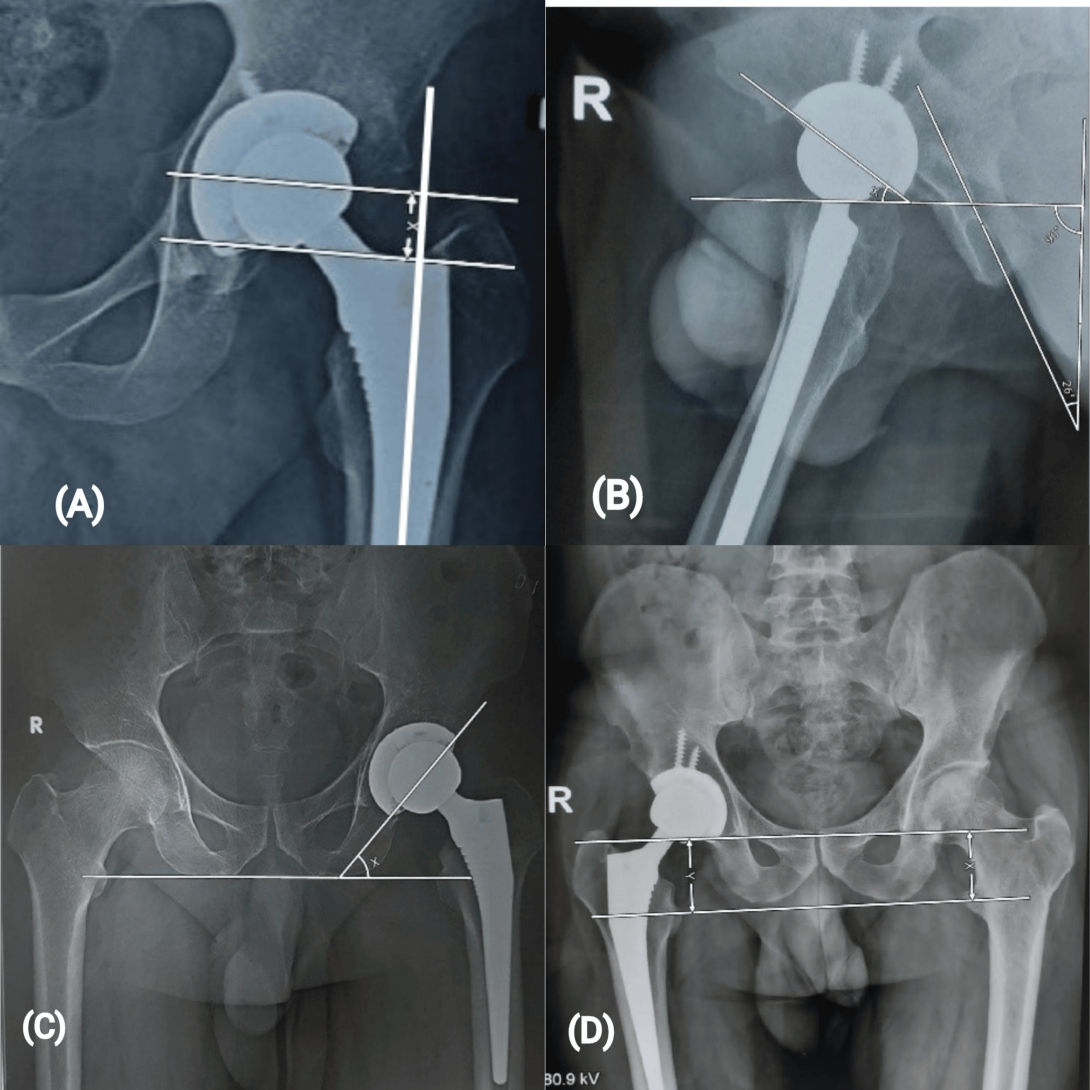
Measurement of (A) Femoral subsidence (x) on anteroposterior (AP) radiograph; (B) Acetabular anteversion (angle x) on cross table lateral radiograph; (C) Acetabular cup inclination (angle x) on AP radiograph; (D) Limb length discrepancy (LLD) (difference between lengths of x and y) on AP radiograph of pelvis
